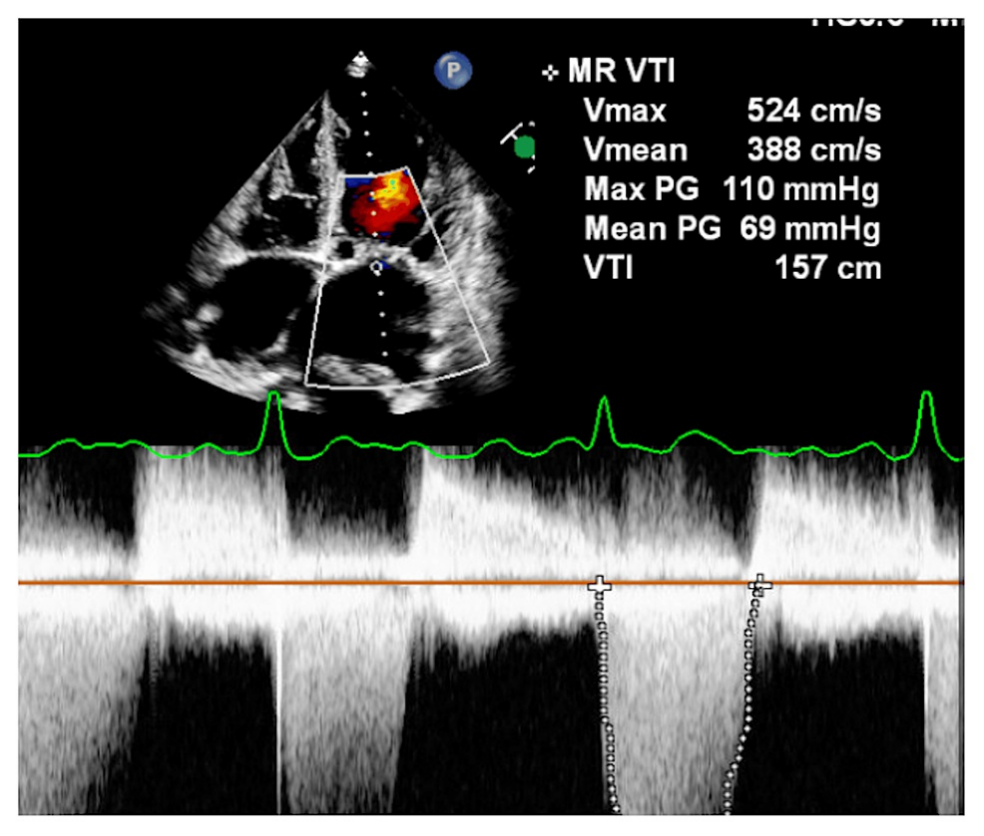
Transthoracic echocardiography continuous wave Doppler showing moderate MS MS: Mitral stenosis
